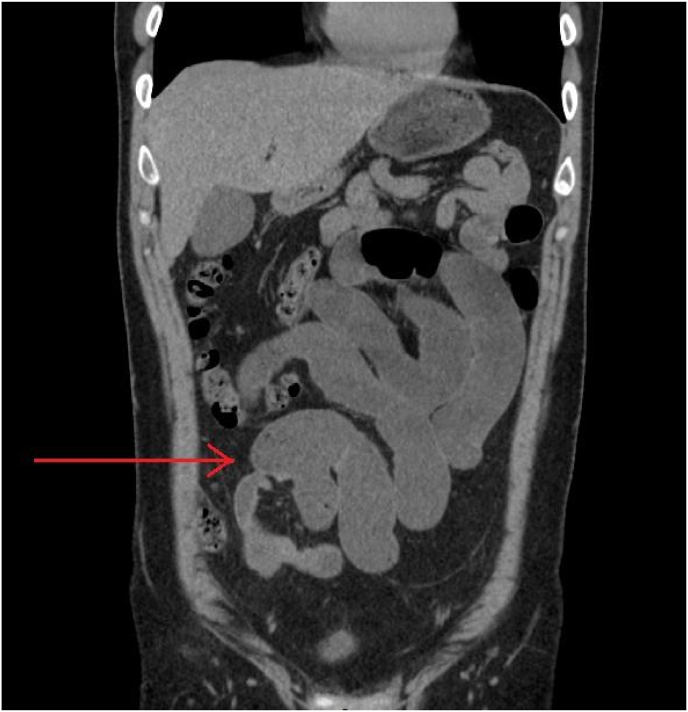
CT scan of the abdomen showing the presence of meckel's loops (red arrow), further alluding to the presence of meckel's diverticulum. (For interpretation of the references to colour in this figure legend, the reader is referred to the Web version of this article.)
